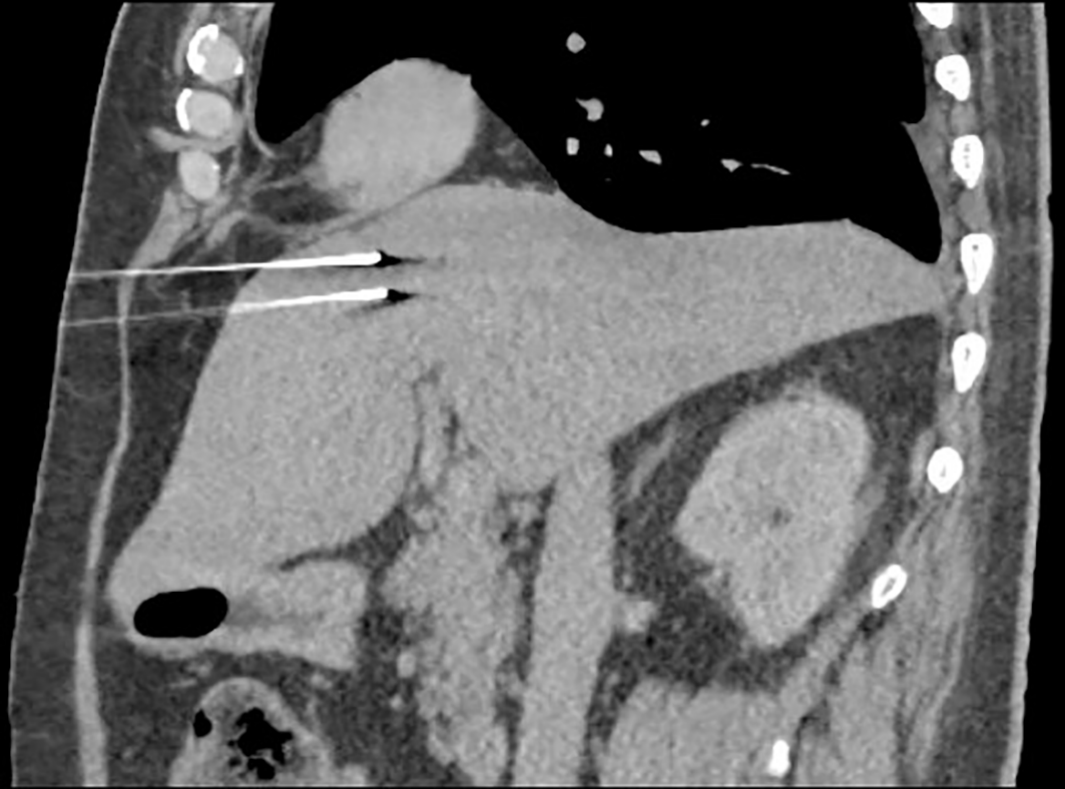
Probe position evaluation performed on CT scan. The current is directed from one probe to the other.
